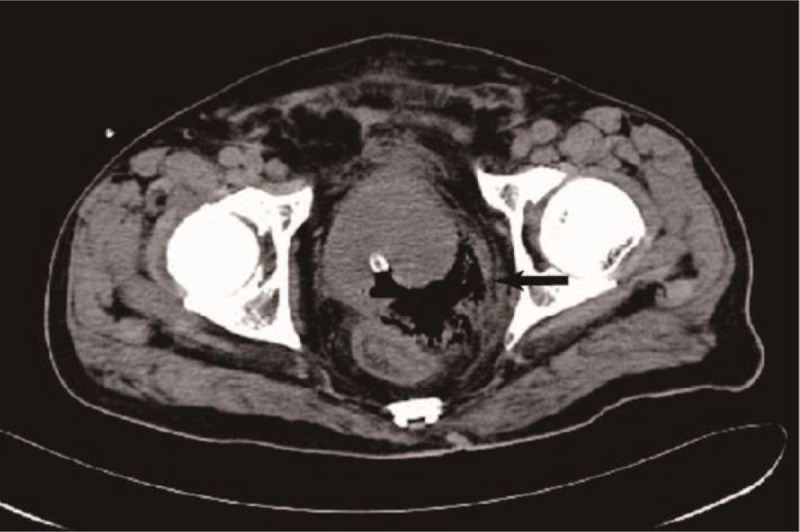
Pelvic CT revealed an improvement of EPA in the prostate gland (arrow) on day 20 of admission (after TUR).
